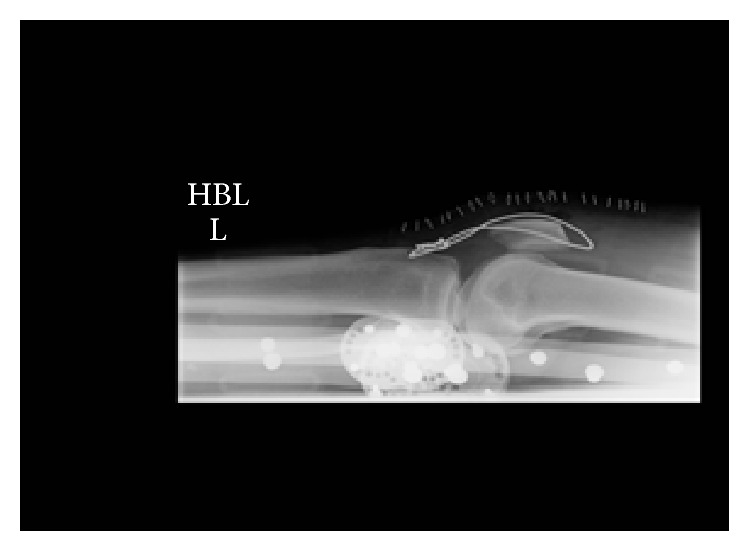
Postoperative left knee radiograph.
